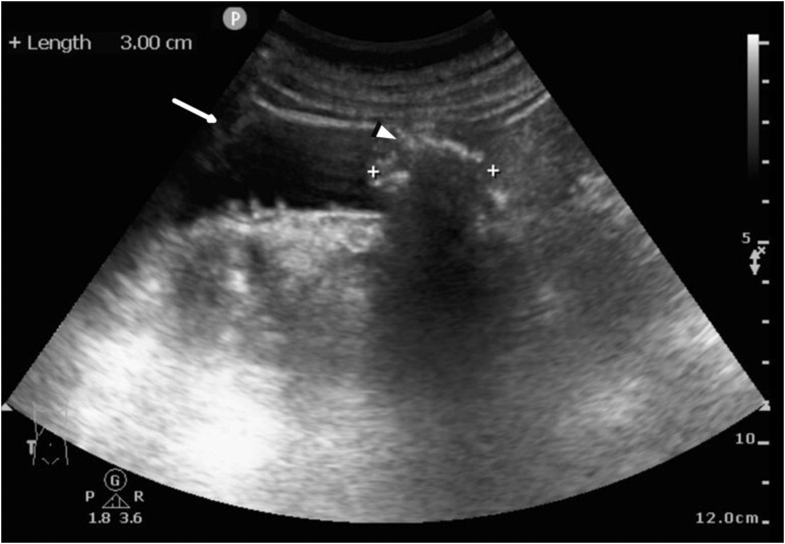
Point-of-care ultrasound demonstrating dilated, fluid-filled small bowel loops (arrow) with intraluminal arch-like hyperechoic lesion (arrowhead).
